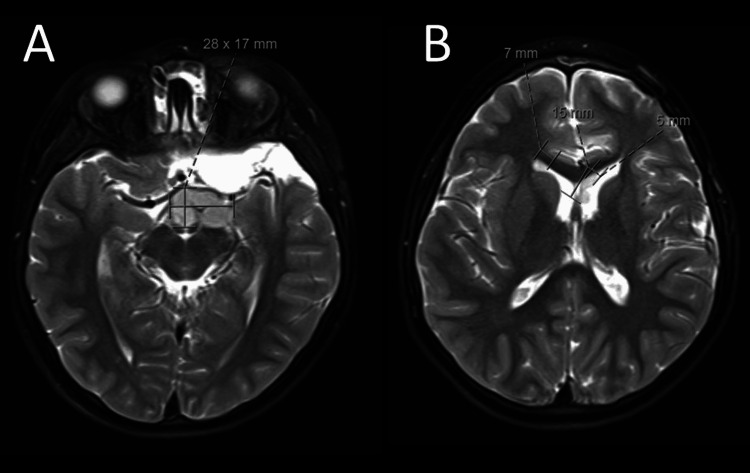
One year post-intervention, brain MRI revealed local tumor recurrence at the suprasellar cistern extending to the interpeduncular cistern and the anterior aspect of the third ventricle. The recurrent mass demonstrates a lobulated irregular margin, but the bulk of the mass is estimated at 27.8 mm x 7.4 mm axially and 12.7 cm craniocaudally at the level of the suprasellar cistern. A portion of the suprasellar component demonstrates diffusion restriction as the initial mass (A). There are nodular foci with similar imaging characteristics with a 7.3-mm nodular focus at the right anterior horn and 15 mm x 4.8 mm nodular foci at the left anterior lateral ventricle abutting the septum pellucidum (B).
